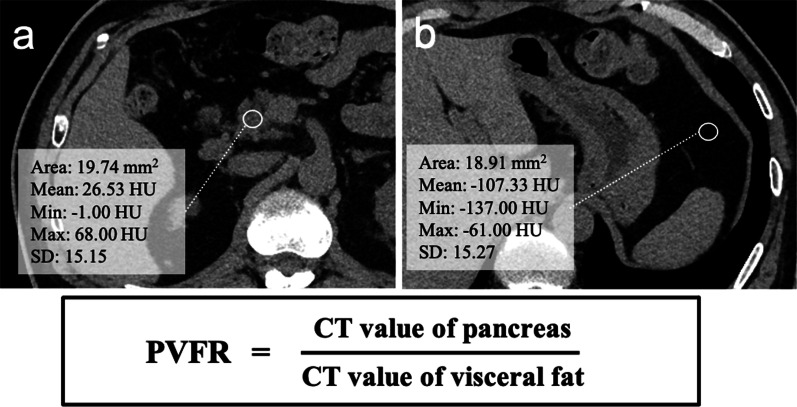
Measurement of the CT value of the remnant pancreatic parenchyma (a) and visceral fat (b). *HU* Hounsfield units, *PVFR* pancreas-visceral fat ratio
